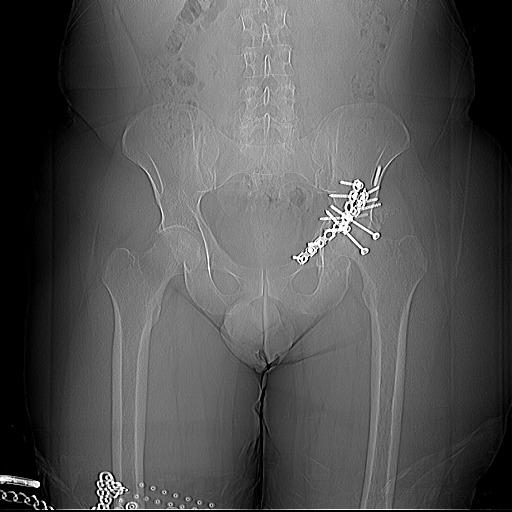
Preoperatory radiography
